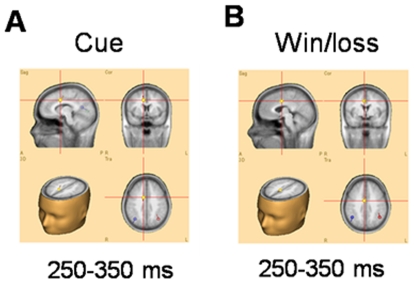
Sagittal, transversal, and coronal views of dipoles. Dipoles were superimposed on MRI-based head models for grand-average ERP waveforms in (A) cue phase and (B) outcome (win/loss) phase.
